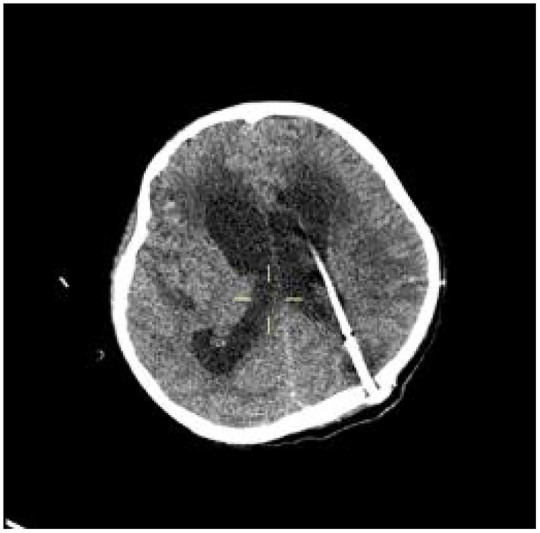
CT demonstrates a left parietal burr hole with the ventricular catheter extending into the anterior horn of the left lateral ventricle.
